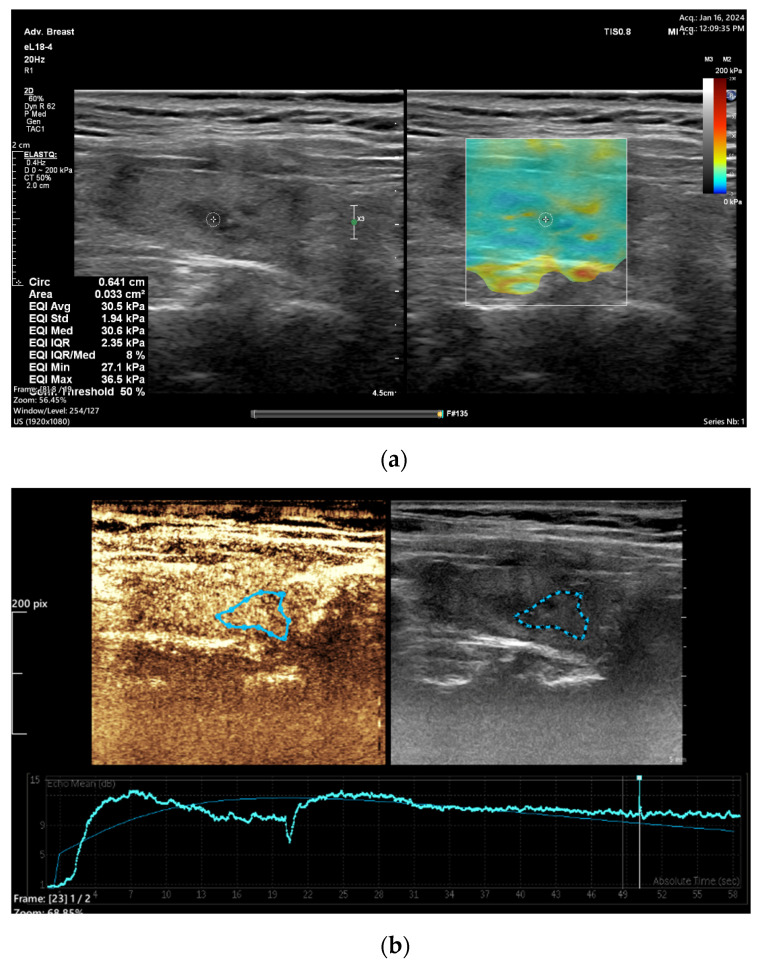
A 66 year old female with papillary thyroid carcinoma overlapped with Hashimoto thyroiditis. (a) Quantitative shear wave elastography (SWE) revealed 30.5 kPa; (b) qualitative contrast-enhanced ultrasound (CEUS) of the nodule revealed homogenous hyper-enhancement and quantitative CEUS revealed a time to peak of 20.3 s and a peak intensity of 11.58 dB.
